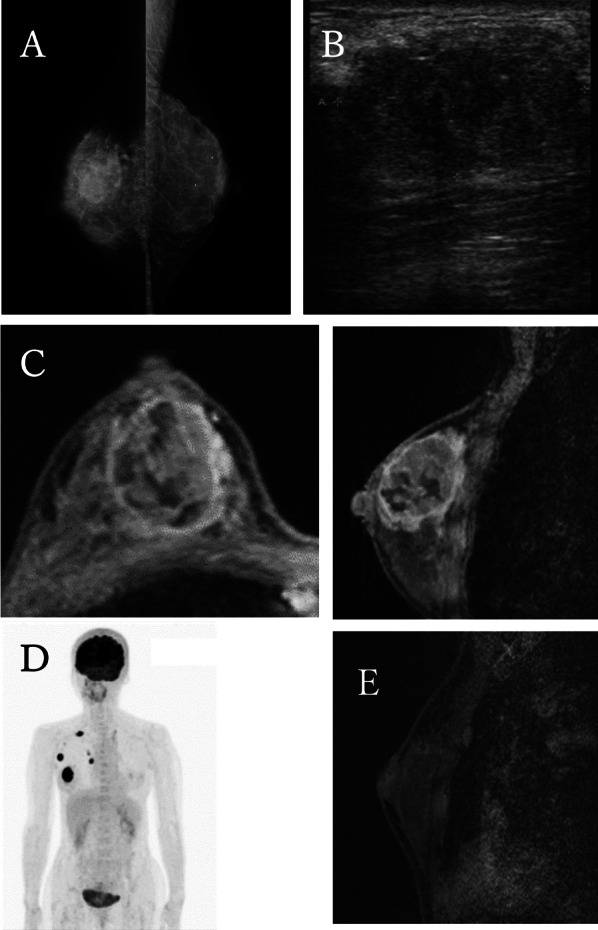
A Mammography revealed a hyperintense mass in the right breast. B Ultrasound showed a 35 mm irregular mass. C Magnetic resonance imaging (MRI) before NAC showed a massive lesion in the right area. The lesion was ring-shaped, contrast-enhancing, with a low-contrast zone. D Fluorodeoxyglucose positron emission tomography (FDG-PET) detected advanced accumulation in the right breast mass, axillary lymph nodes, right supraclavicular lymph nodes and right internal thoracic lymph nodes. E MRI after NAC showed a massive lesion was significantly reduced
